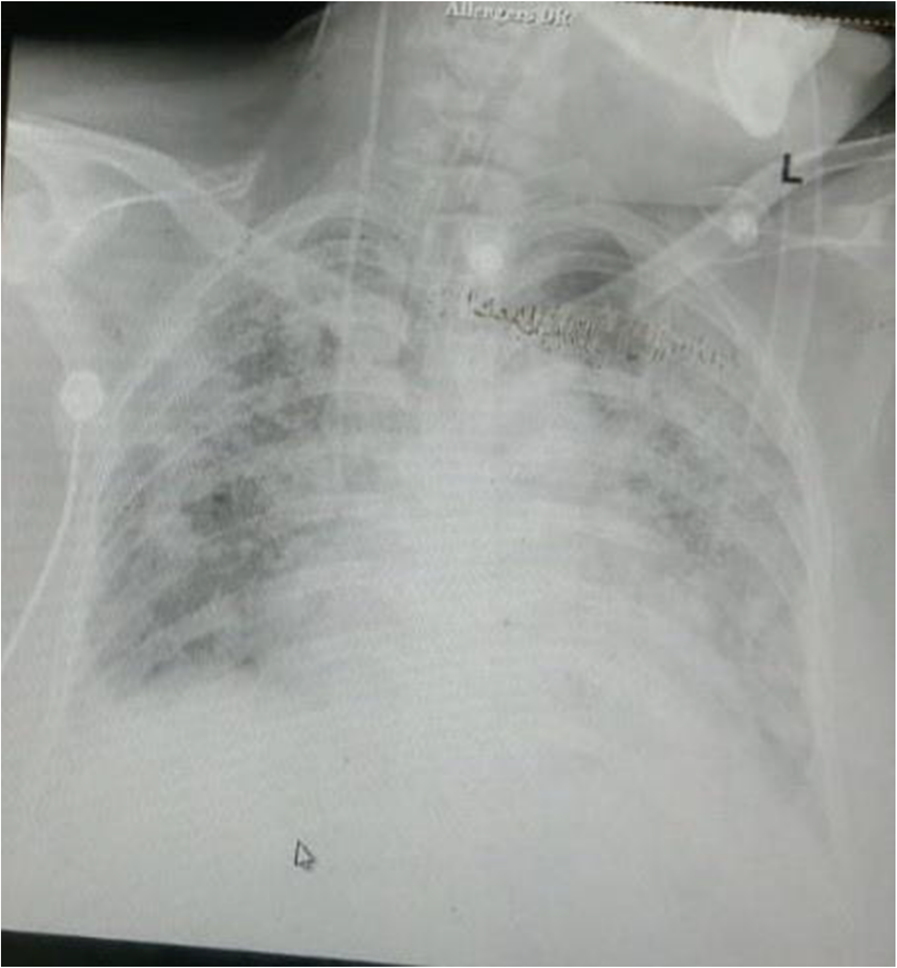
Chest X-ray on POD6 showing acute congestive cardiac failure with ARDS
